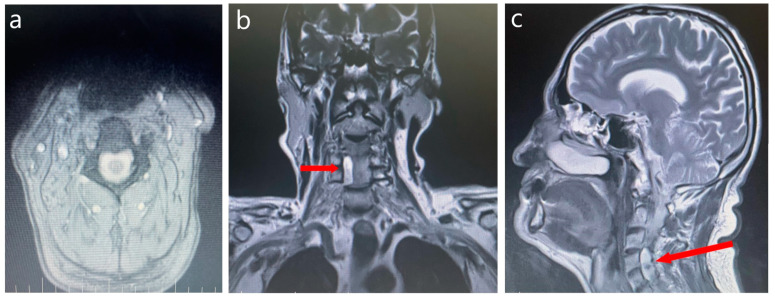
Cerebral and cervical spine MRI: axial (a), coronal (b) and sagittal (c) plane. The red arrows show the presence of the arachnoid cyst at the level of the C4–C5 segment.
